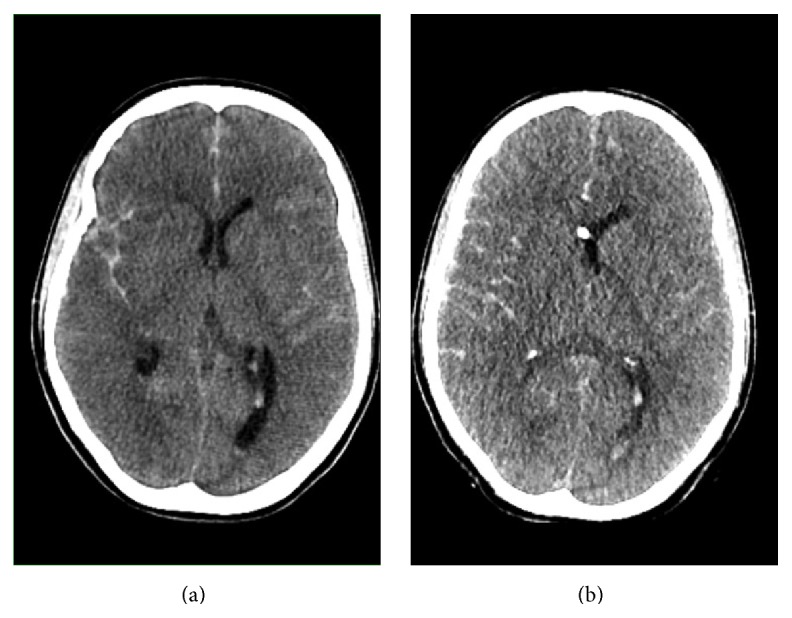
(a) First computed tomography of the head (01:25 am) and (b) follow-up computed tomography of the head (02:30 am).
